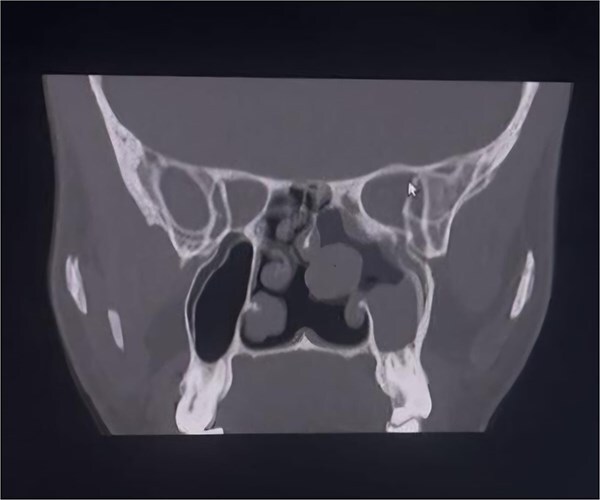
Coronal CT PNS (bone window) soft-tissue mass involving the left nasal cavity with associated left maxillary sinus opacification/extension and sinonasal involvement consistent with recurrent JNA spread.
